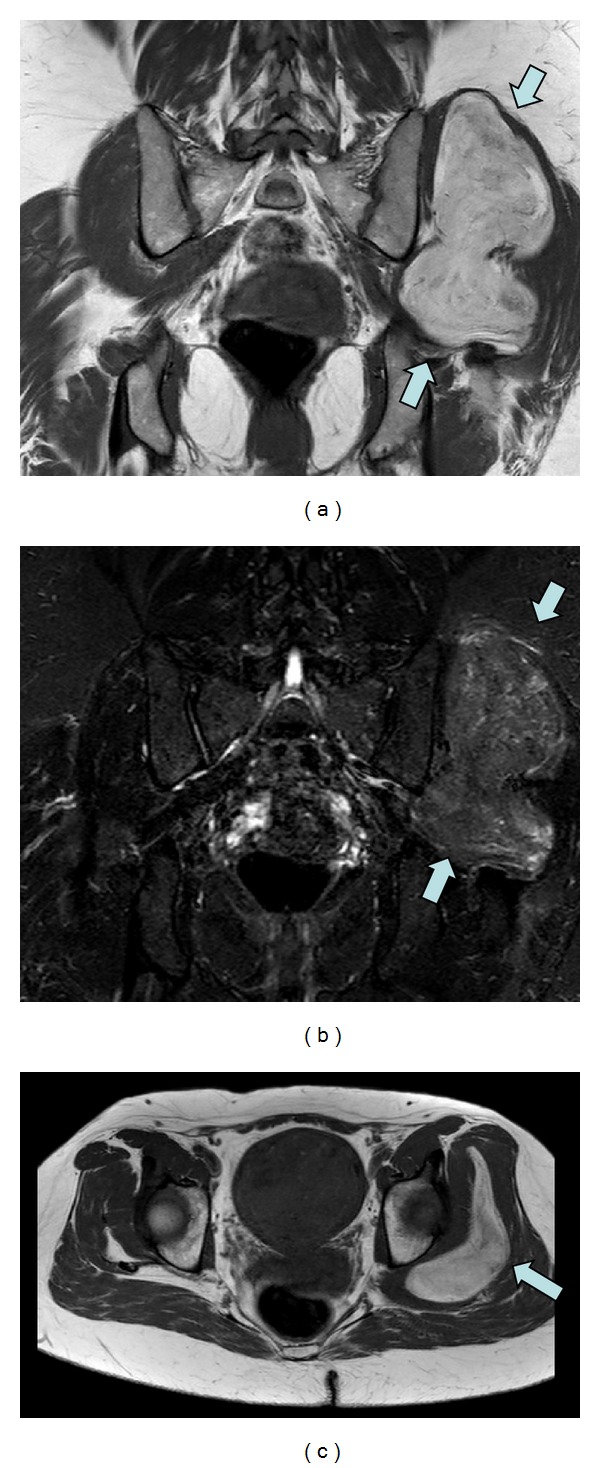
(a) Coronal T1, (b) coronal STIR, and (c) axial T1 MRI images demonstrate a lobulated mass of complex fat attenuation arising from the left gluteus medius muscle, outlined by blue arrows.
